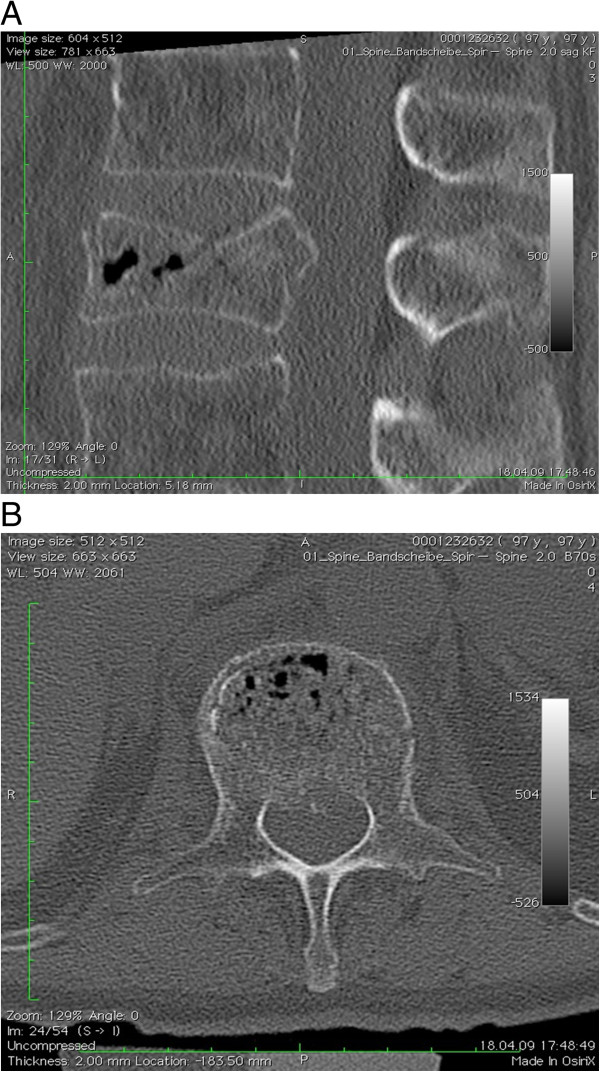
A to 2-C CT scan of the spine of the 97-year-old patient who presented with an incomplete motoric paraplegia (Frankel grade C) associated with an L 2 burst fracture. A Preoperative sagittal reconstruction. B Preoperative axial CT scan showing 50% canal compromise. The patient was managed with near-anatomic reduction without any direct reduction of the fracture fragment.
